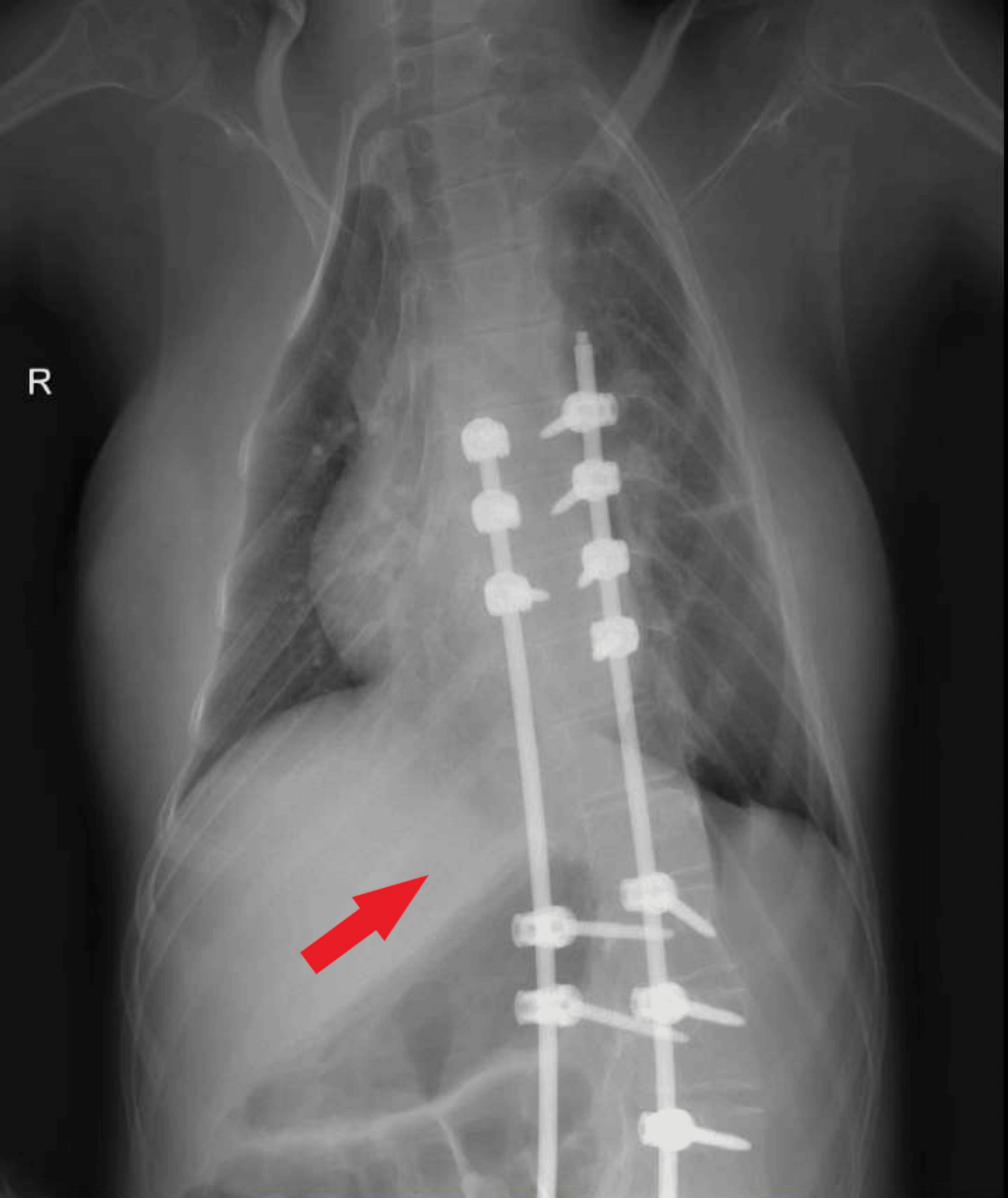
Postoperative chest X-ray of the patient shows reduction of the deformity.
